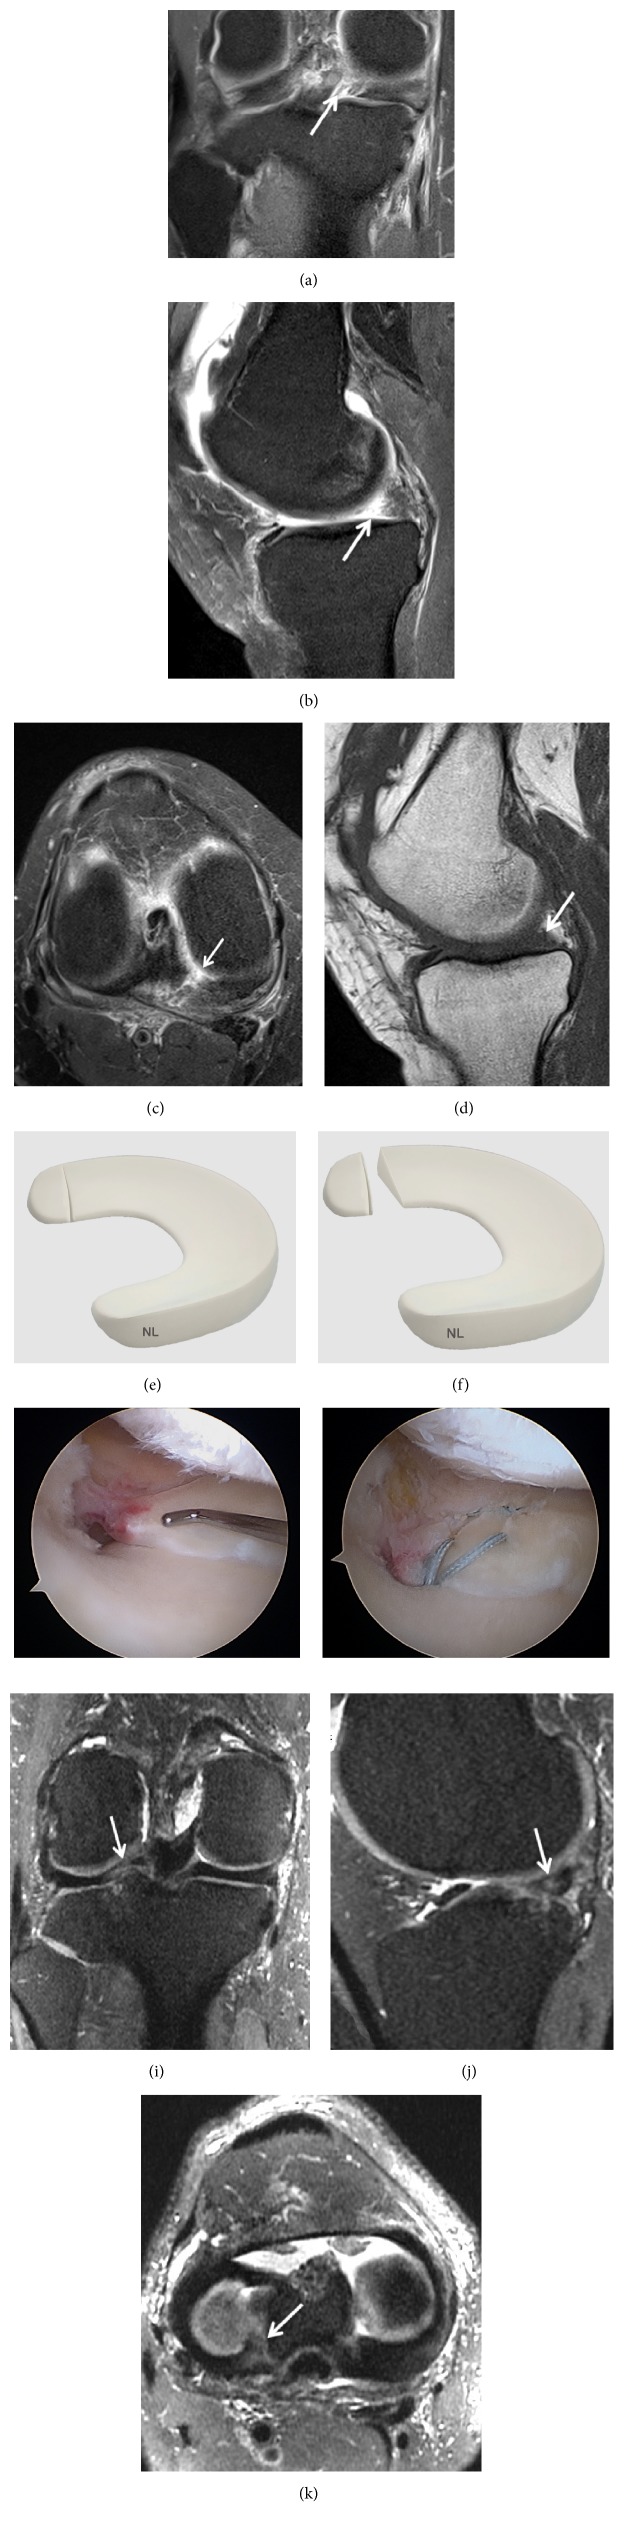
Meniscus posterior horn avulsion. (a) T2-weighted fat-saturated images showing a complete posterior root tear of the medial meniscus (arrow); (b) Ghost meniscus sign. The posterior horn of the medial meniscus has been replaced with triangular high signal intensity on the T2-weighted fat-saturated sequence (arrow); (c) axial reconstruction showing the large posterior horn avulsion (arrow) with high signal intensity on the T2-weighted fat-saturated sequence; (d) the posterior horn of the medial meniscus is not identified on the sagittal T1 (arrow); (g) arthroscopic view showing a displaced medial meniscus root tear; (h) arthroscopic view showing a suture of the medial meniscus root tear; (i) identification of root tears of the lateral meniscus can be more difficult on the coronal T2-weighted fat-saturated sequence; (j) ghost meniscus sign is less significant on the sagittal T2-weighted fat-saturated sequence (arrow); (k) axial reconstruction showing the posterior horn avulsion (arrow).
